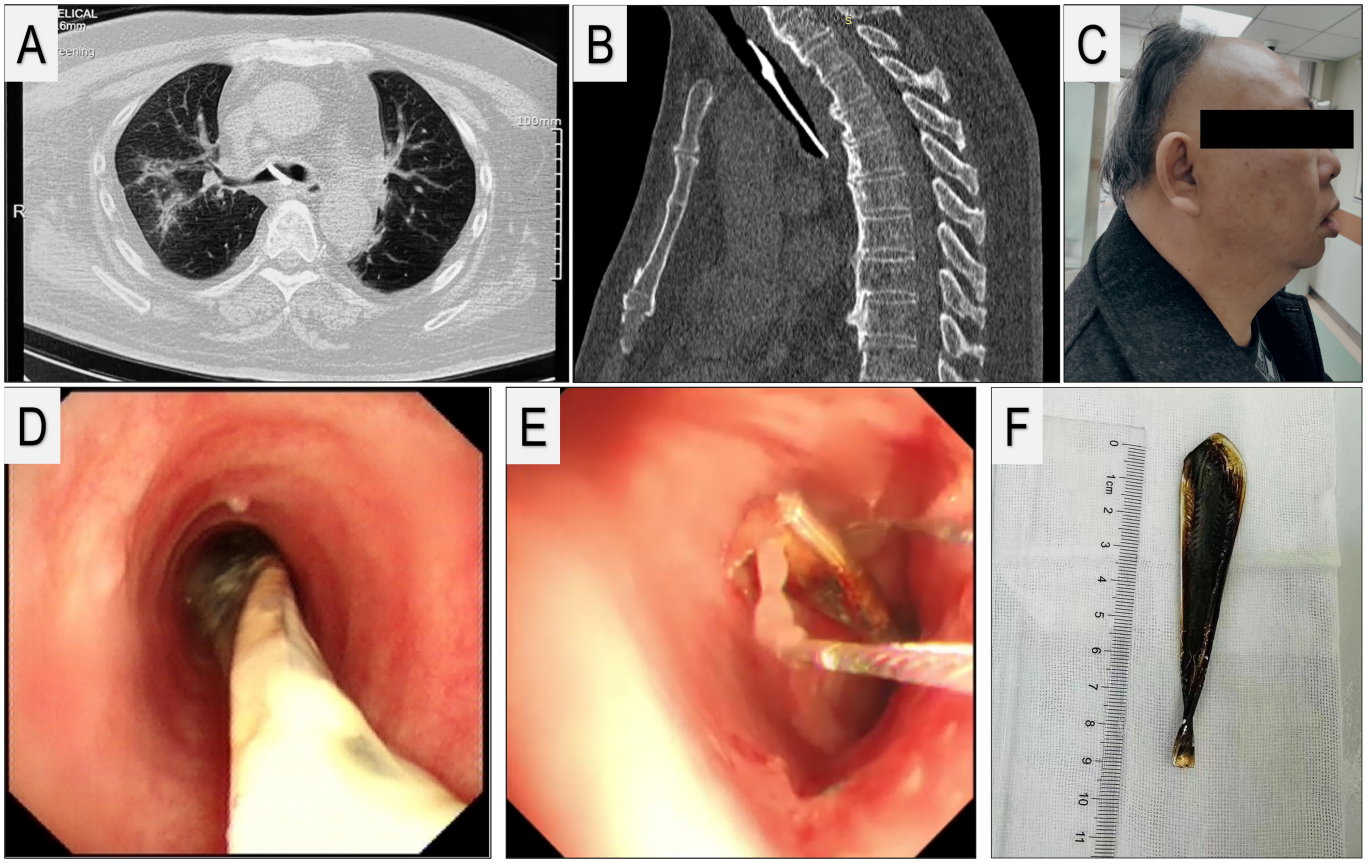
(A) CT scan of the chest showing the lower end of the foreign body was inserted into the right main bronchus and bronchopneumonia lesion. (B) A sagittal plane of the CT scan of the chest showing the location of the foreign body in the trachea. (C) Right-side view of the patient's facial appearance, accompanied by the Mallampati score IV and thyromental distance: 3 fingerbreadths. (D) Initial bronchoscopy view of the foreign body occluding the trachea. (E) Ligation of the upper segment of the foreign body with a snare via flexible bronchoscopy. (F) The foreign body, a metal spoon handle, ~10 cm long.
